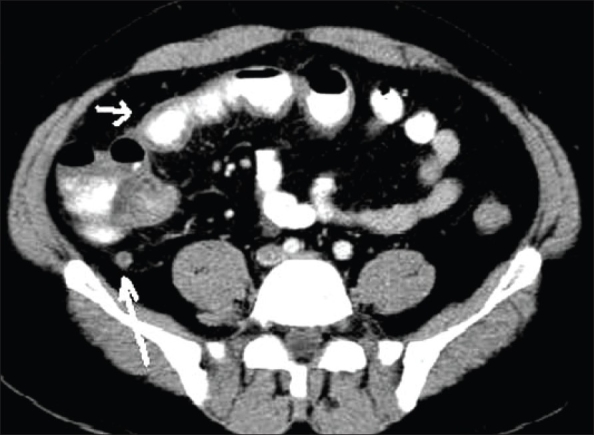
Crohn's disease. Contrast-enhanced CT scan shows distal ileal loops with long segment circumferential wall thickening (short arrow) and adjacent fat stranding and skip lesions. The normal appendix is also seen (long arrow)
